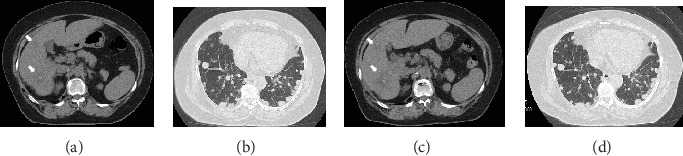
Abdominal and chest CT before the administration of S-1 (a, b). Stable disease after therapy (c, d). Arrows: lesions in the liver.
